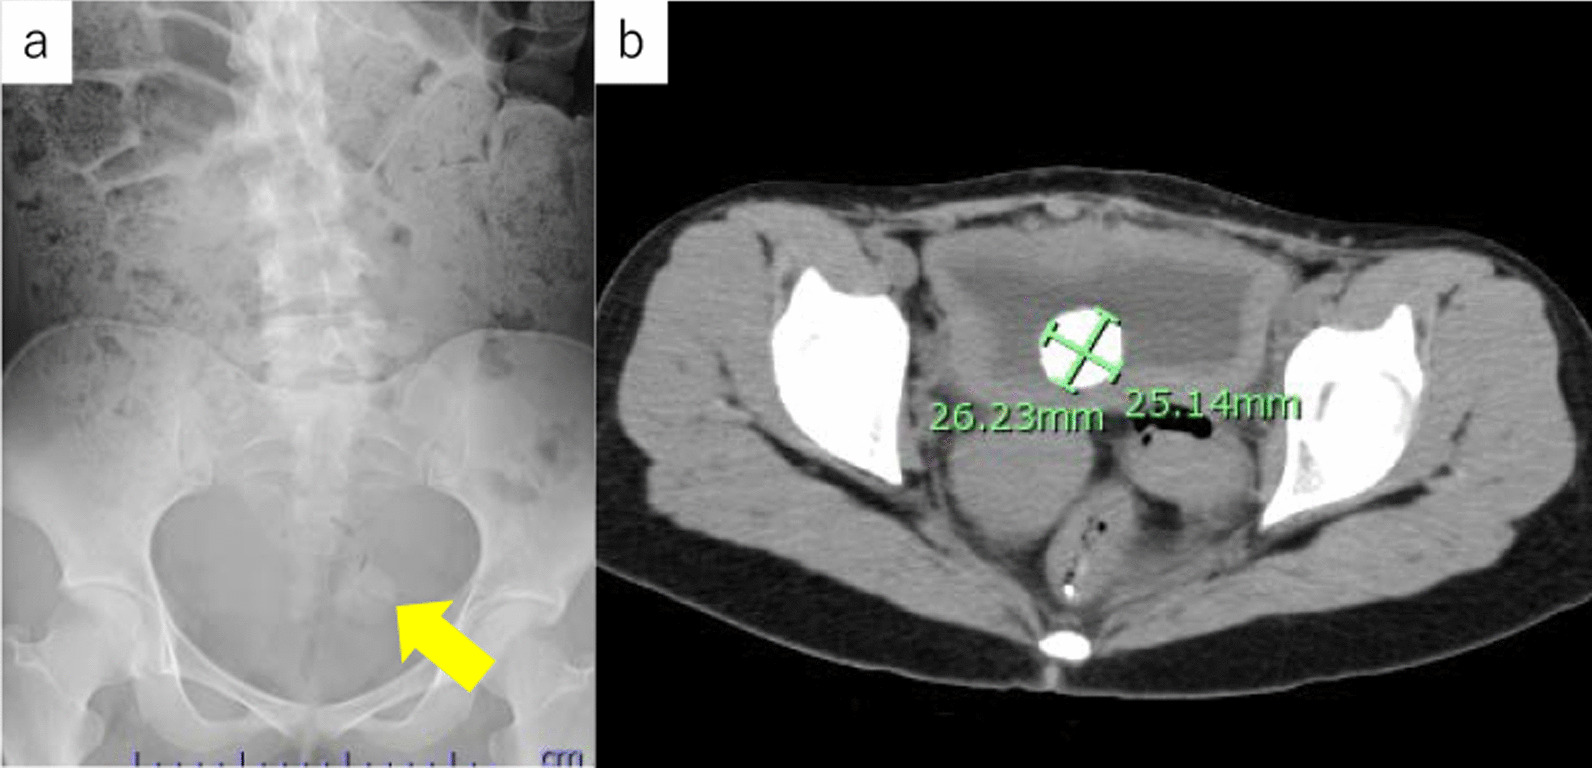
Preoperative a KUB and b CT (target stone: yellow arrow)
